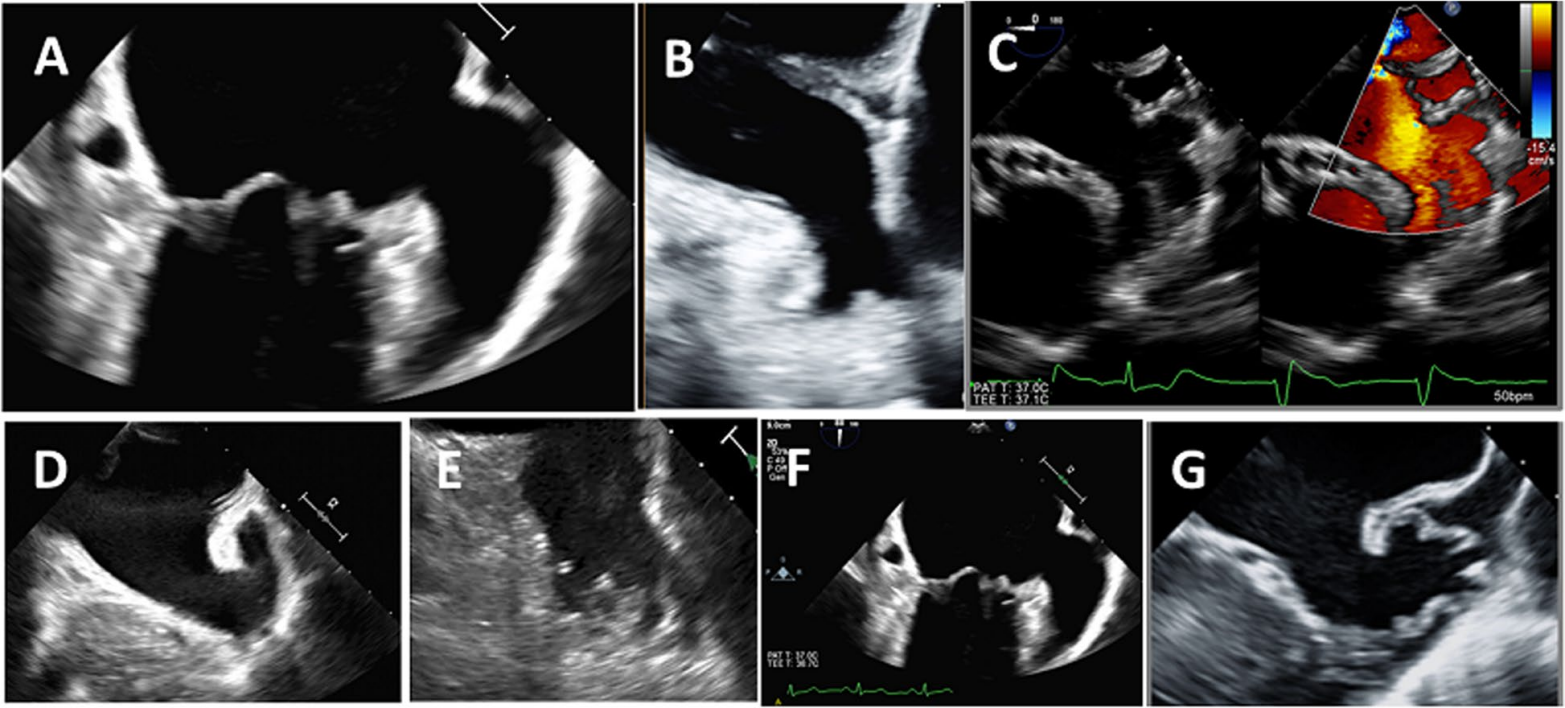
Upper panel shows two-dimensional transesophageal echocardiography examples of the left atrial appendage (LAA) lobe. A Single-lobed, (B) bi-lobed, an (C) tri-lobed LAA with color Doppler flow inside. Lower panel shows different LAA morphology of (D) chicken wing, (E) cactus, (F) windsock, and (G) cauliflower
